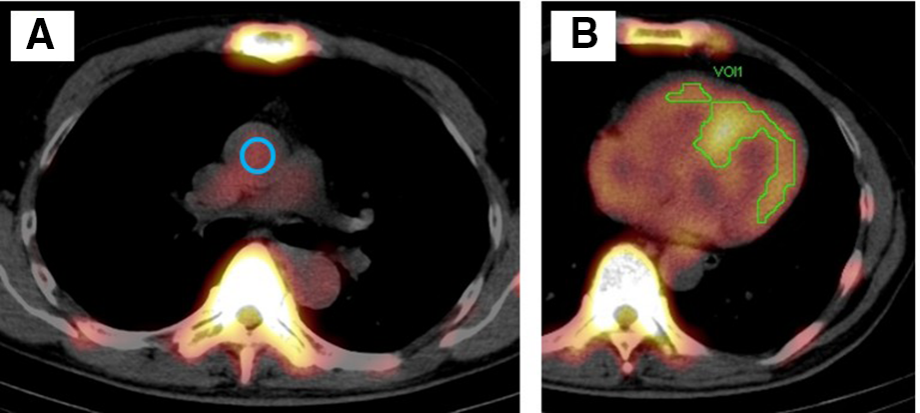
An example of SUV-thresholded volumetric evaluation. The ^99m^Technetium-pyrophosphate (^99m^Tc-PYP) activity was shown as an overlay on the computed tomographic imaging. A spherical volume of interest (blue circle, a) was placed in the center of the ascending aortic blood pool (ABP) at the height of the pulmonary artery bifurcation. The myocardial regions where ^99m^Tc-PYP uptakes were > 1.2 × ABP radioactivity (SUVmax) were automatically evaluated using xSPECT Quant (green contour, b). SUV, standardized uptake value
